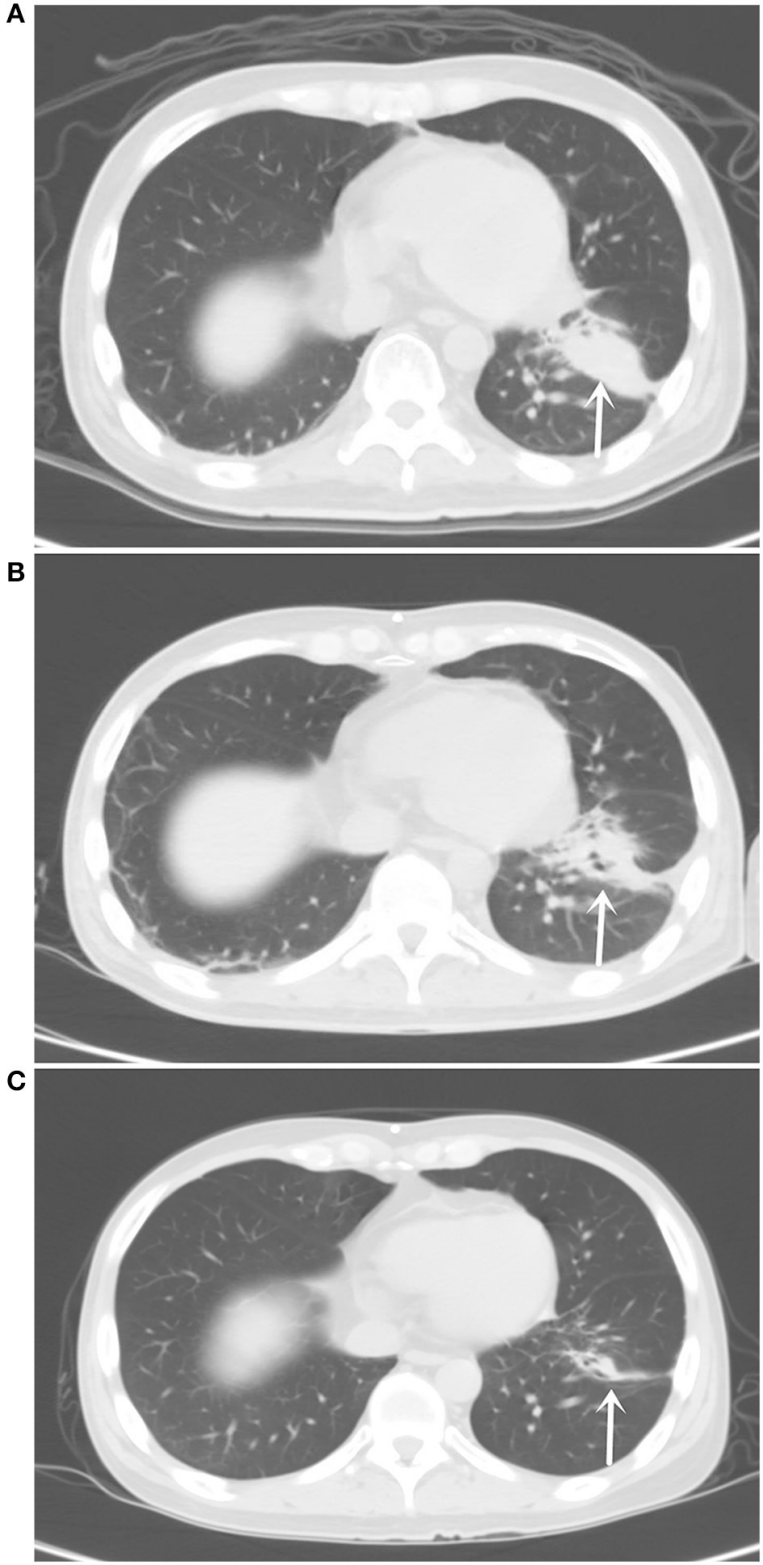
(A) Chest computed tomography (CT) pulmonary window on April 19, 2018 showed an irregular solid mass in the lower lobe of the left lung of about 4.0 × 1.9 cm in size. (B) Chest CT pulmonary window on July 25, 2018 showed the tumor in the lower lobe of the left lung with an irregular shape and uneven density at about 3.5 × 1.1 cm. (C) Chest CT pulmonary window on September 18, 2019 showed an irregular solid mass striated shadow in the lower lobe of the left lung at 2.1 × 0.4 cm in size.
